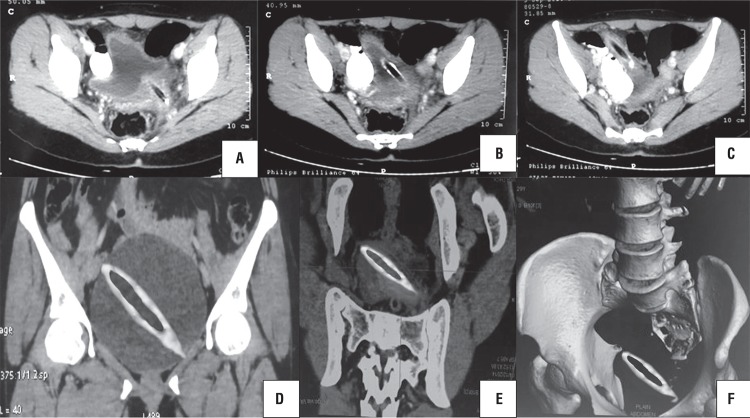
Computed tomography scan [axial section (A-C) and coronal section (D-F)] showing hypodense linear foreign body (10.2 x 1.2cm) piercing the anterior vaginal wall and left posterior bladder wall with majority of its part lying inside the bladder. The tip of foreign body pierced right to anterior bladder wall and reached the abdominal wall.
